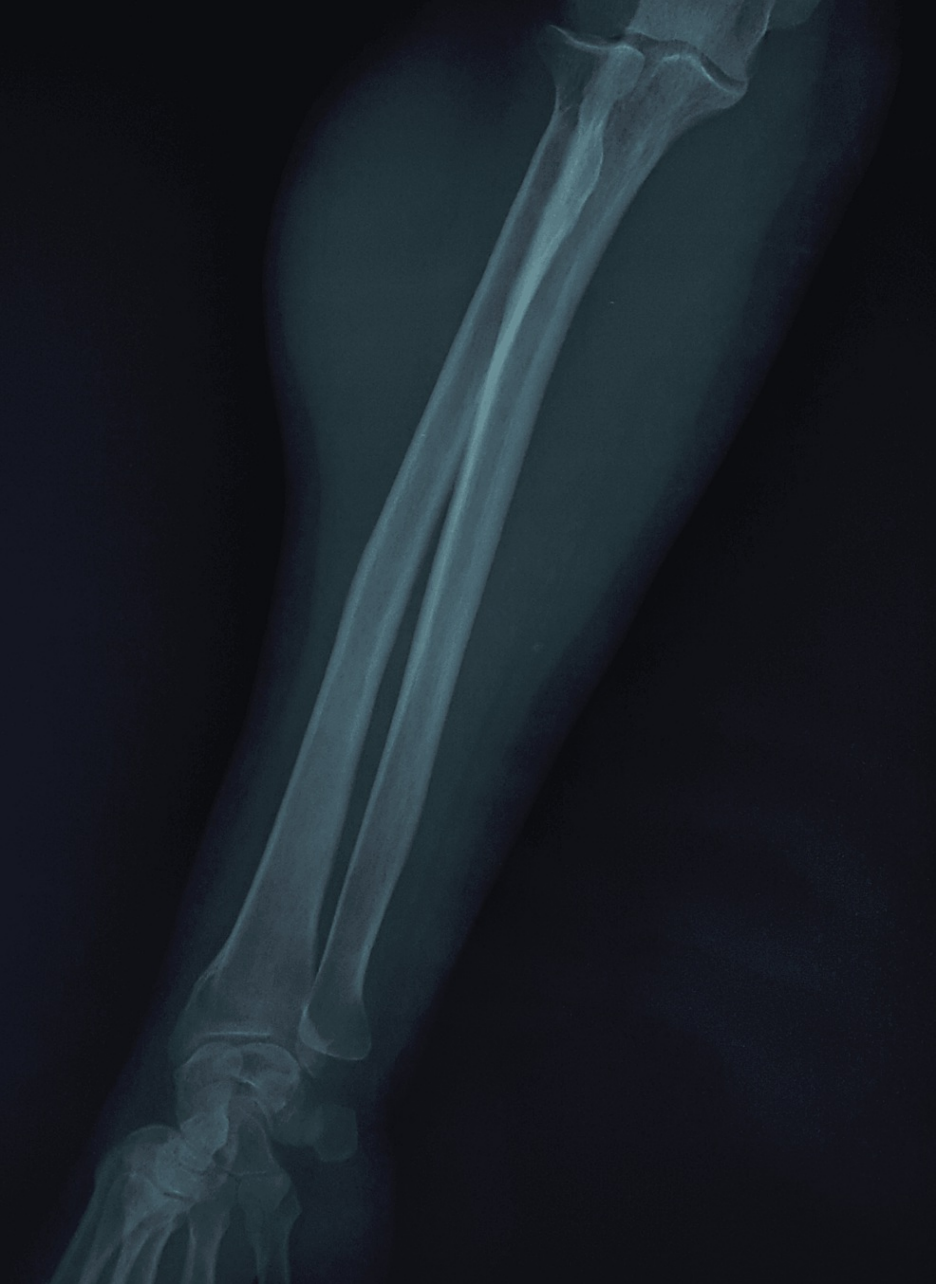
Anteroposterior X-ray view of the right forearm
